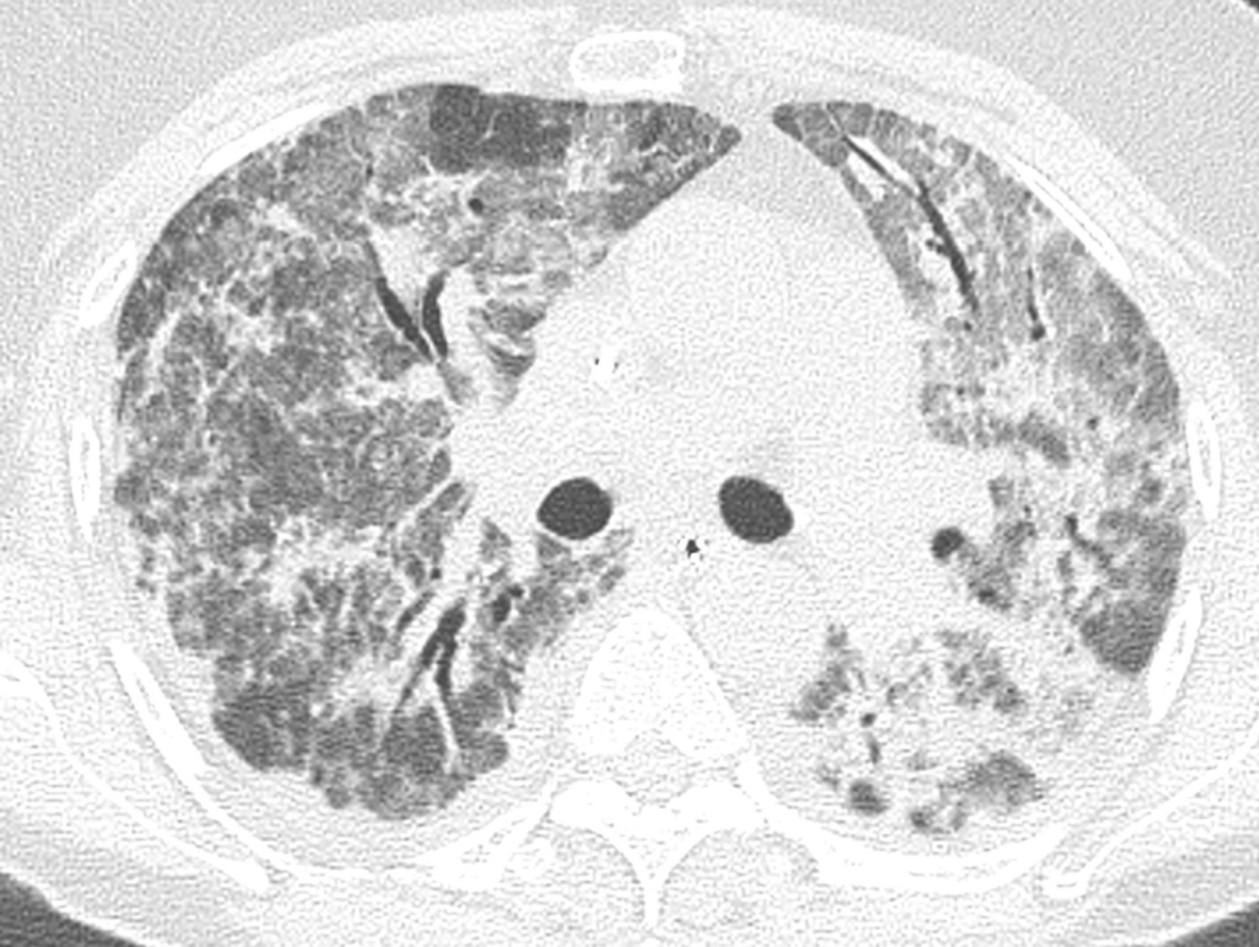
Air bronchogram. Axial reconstructions of a CT in lung window showing air bronchogram.
